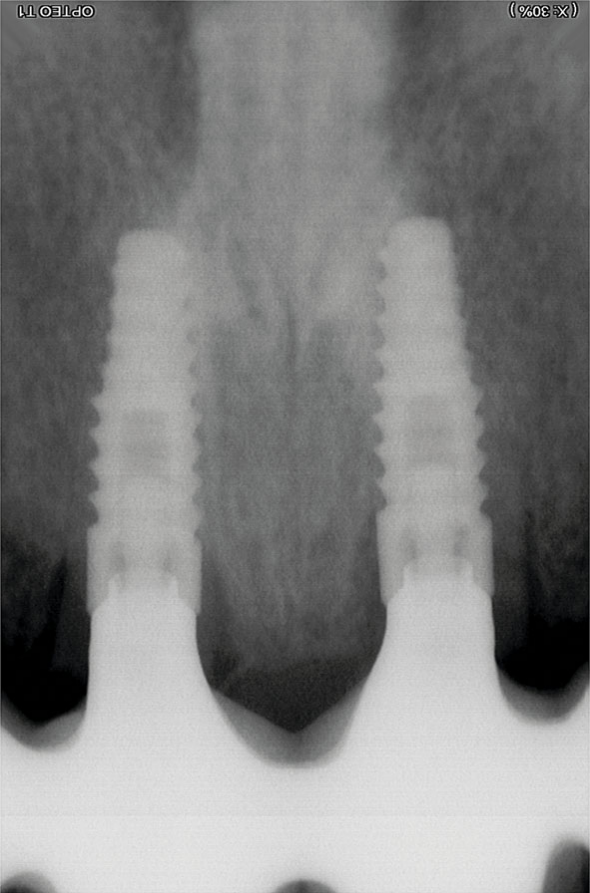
Control X-ray after bridge placing.
